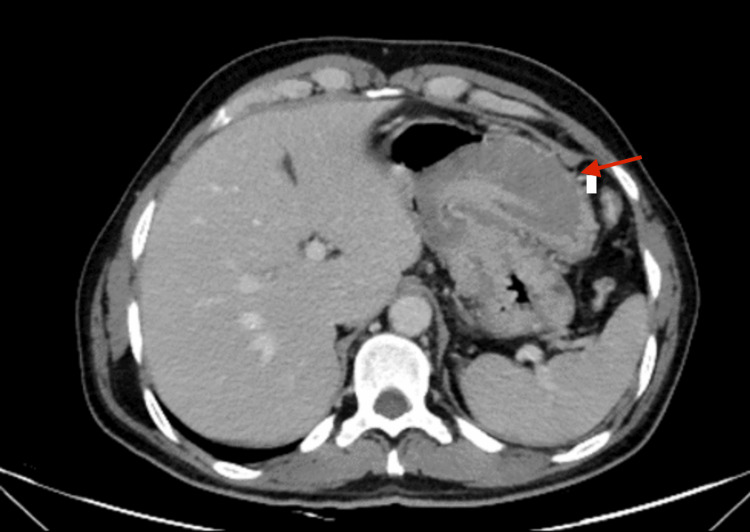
Abdominal CT scan Gastric wall thickening at the proximal greater curvature (arrow). CT: computed tomography
